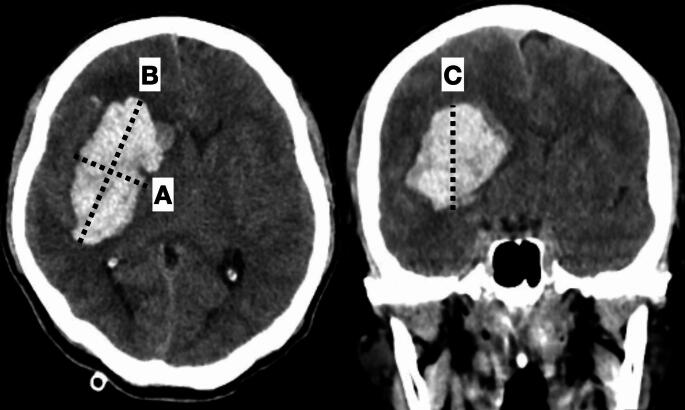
Volume of the hemorrhage A x B x C/2. A dash line, the greatest hemorrhage diameter on the CT axial view; B dash line, the diameter perpendicular to A dash line; C dash line, the maximal diameter of the hemorrhage on the coronal view
